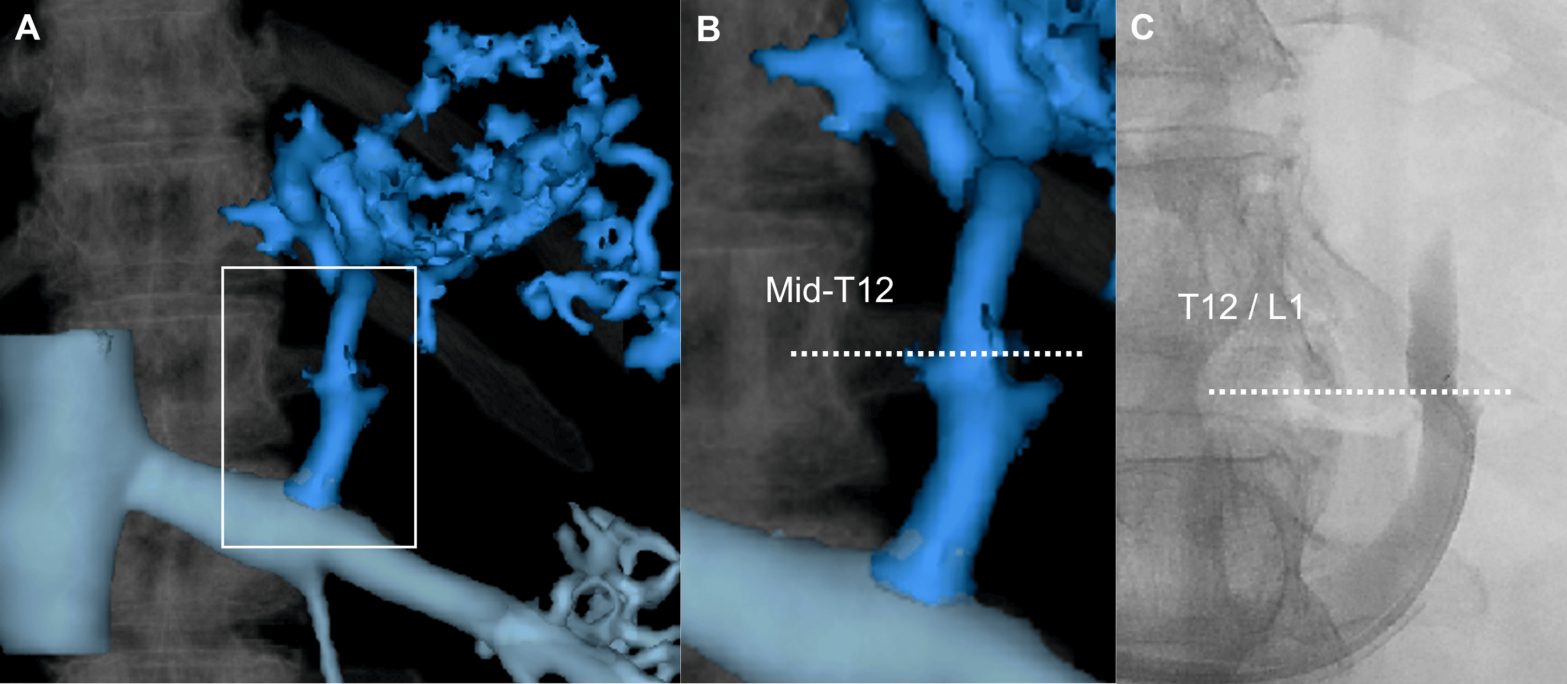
Discrepancy in the vertebral level of the stenosis between 3DCT portography and intraoperative venography A: 3DCT portography image of gastric varices, including the GRS, left renal vein, inferior vena cava, and vertebral bodies. The GRS outlet is highlighted by a rectangle to indicate the region of interest. B: 3DCT portography image showing the stenosis of the GRS outlet located at the mid-T12 vertebral level. C: Intraoperative venography image showing the corresponding stenosis at the T12/L1 intervertebral level. GRS: gastrorenal shunt; 3DCT: three-dimensional computed tomography
